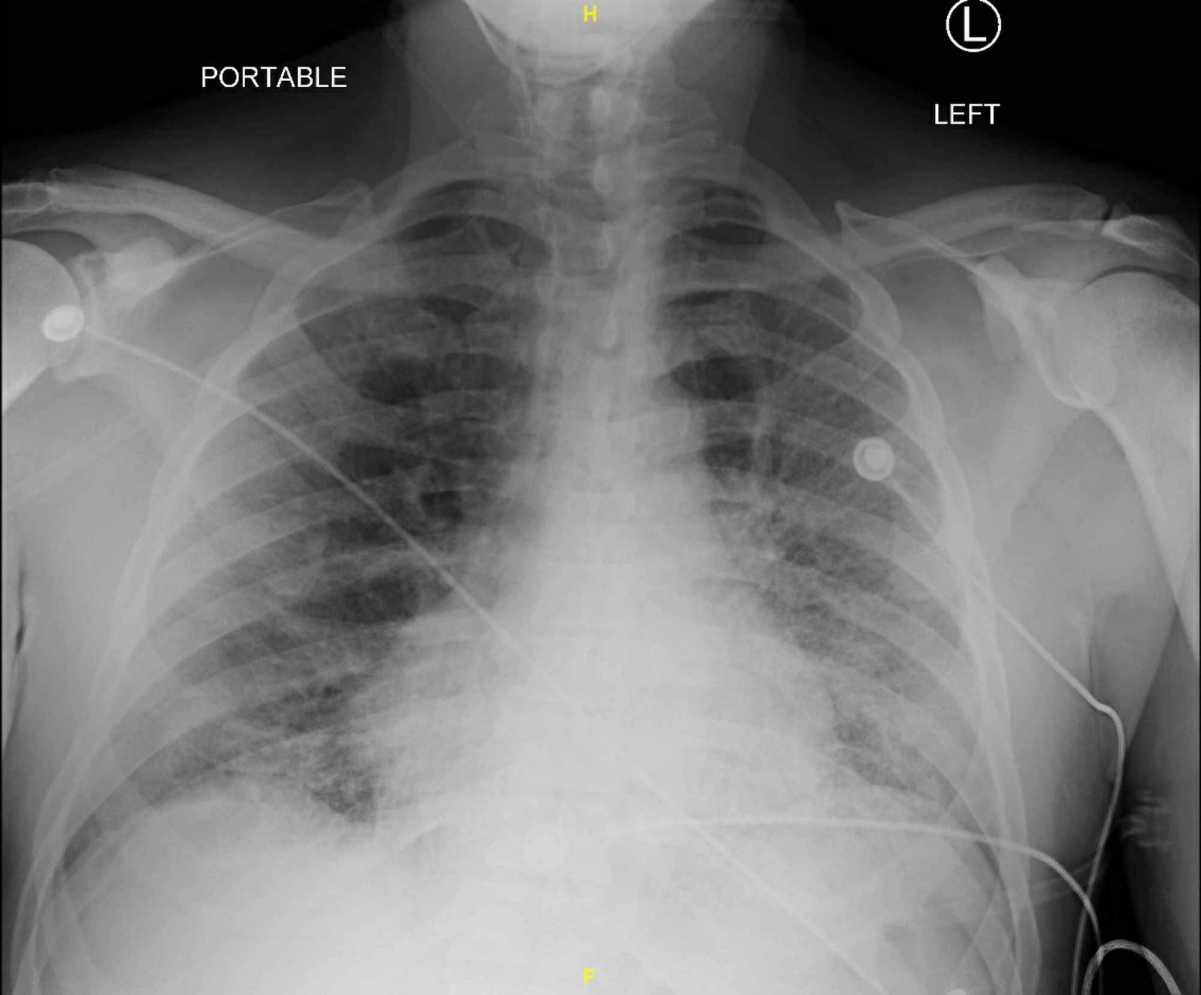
Posteroanterior (PA) chest X-ray upon admission Demonstrating bilateral pulmonary consolidations, more extensively on the left. Findings compatible with viral pneumonia.
